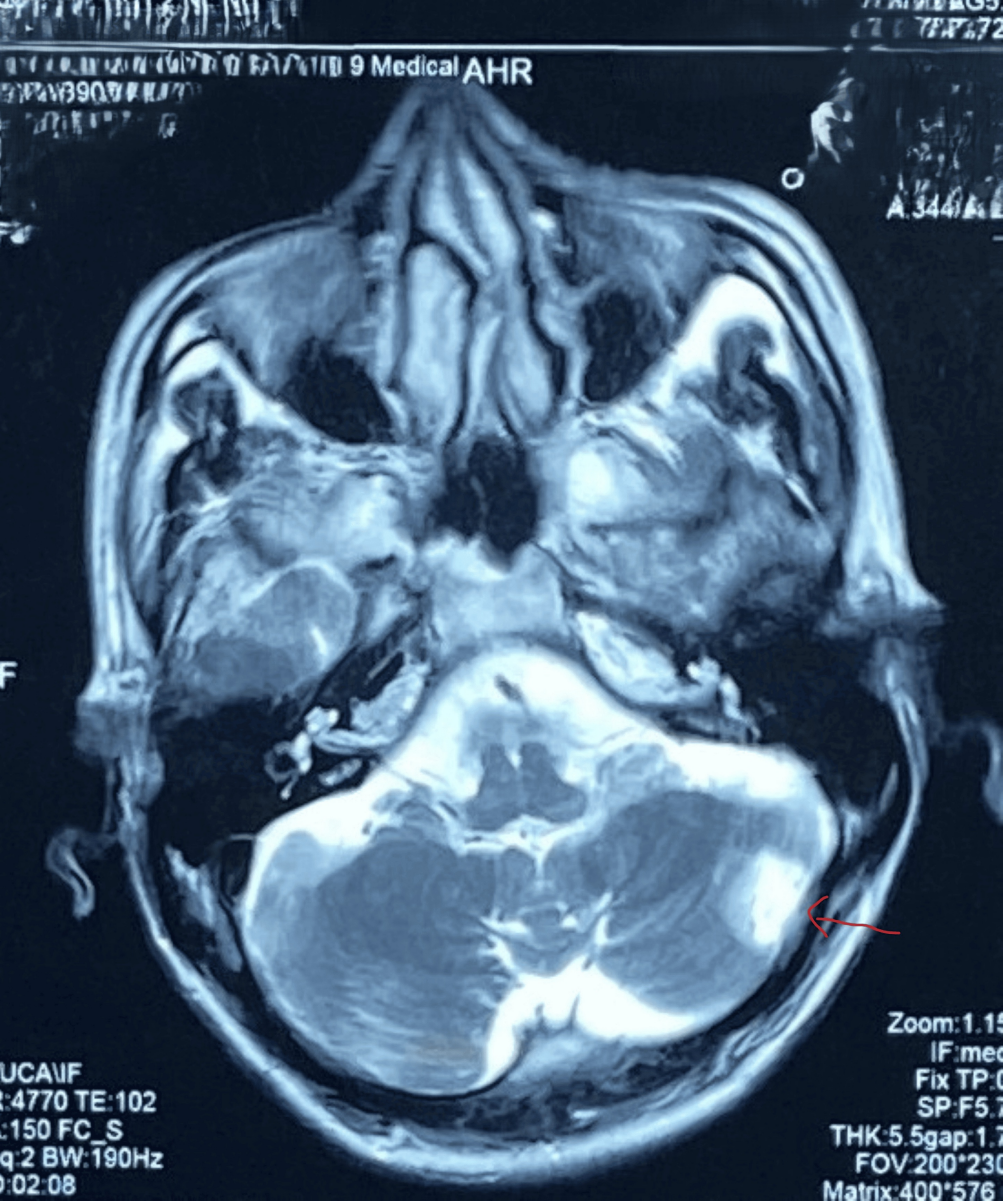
Axial T2-weighted MRI of the brain. The arrow showa a focal area of signal intensity in the left cerebellar hemisphere.
